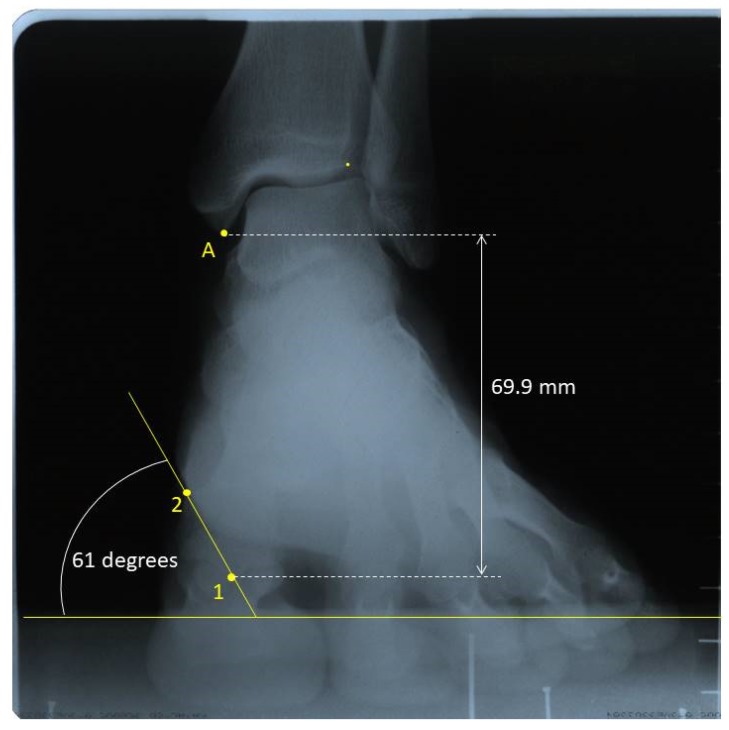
Radiographic measurements in one foot with first ray in neutral position. Point 1: intersesamoid crest; Point 2: superomedial tubercle of the first metatarsal head. Point A: the most distal tip of the tibial malleolus.
